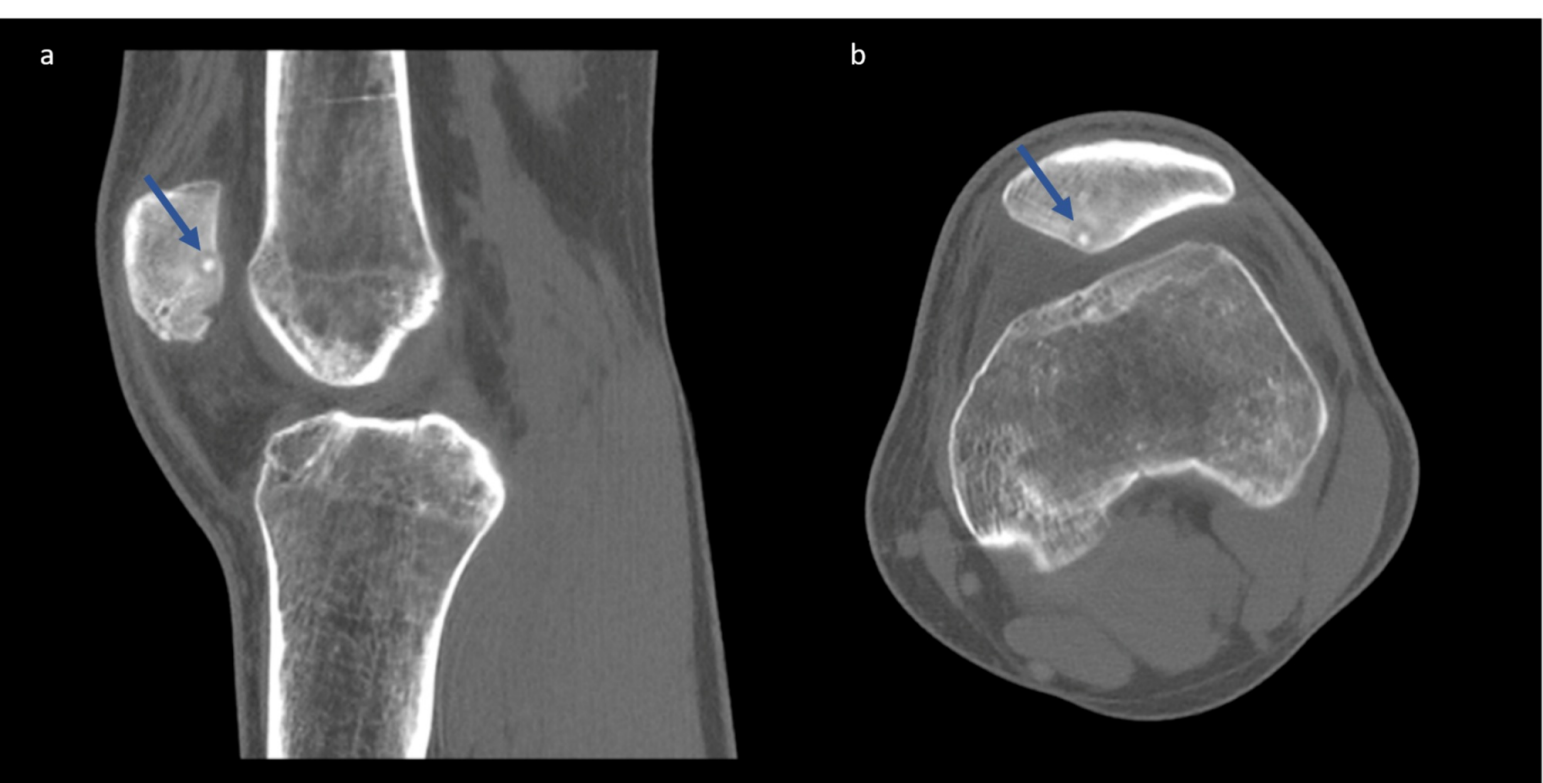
CT scan reveals the pathognomonic nidus appearance Blue arrows: characteristic nidus on a subchondral location
